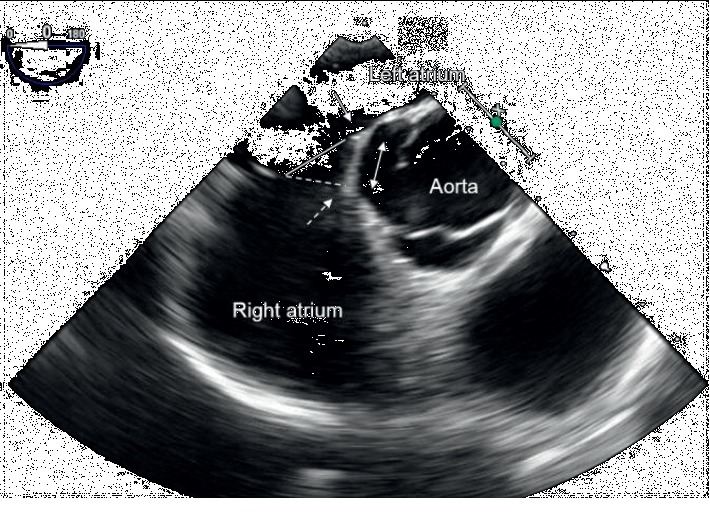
Transesophageal echocardiography showing septal malalignment. The septum primum attached to the aorta is malaligned toward the left atrial side (solid arrow). The septum primum is separated from the septum secundum (dotted arrow). The defect surface of the septum primum (solid line) is different from that of the septum secundum (dotted line). The distance of separation between the septum primum and the septum secundum is measured (double arrow).
